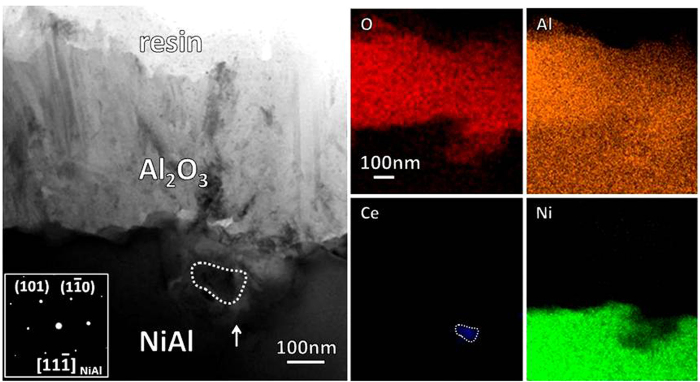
STEM BF image and corresponding elemental X-ray mappings of the cross-sectioned Al_2_O_3_ scale on the aluminide after 5 min oxidation at 1100 °C.
